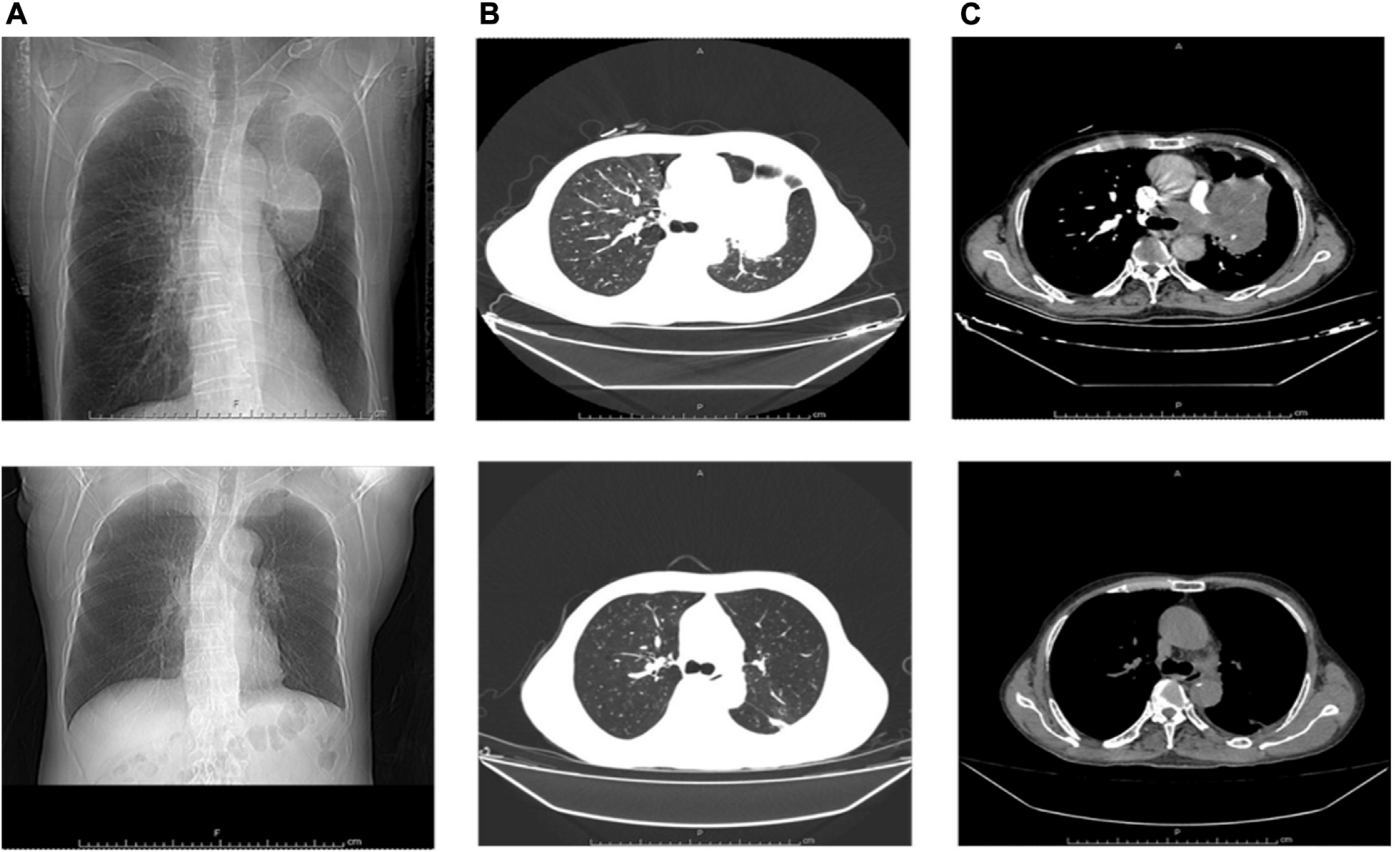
Comparison of lung CT scan before and after chemotherapy. (A–C) Before chemotherapy; (A–C) After chemotherapy; (A), (a) Frontal; (B), (b) Lung Window; (C), (c) Mediastinum Window.
